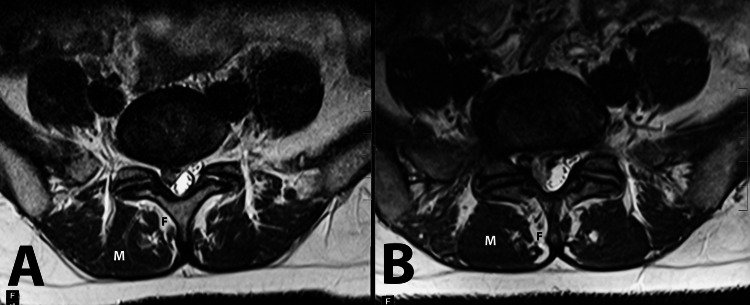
Axial T2-weighted MRI images of Patient 2 at initial presentation (A) and three years later. Note the progression of fatty infiltration (black letter F) and multifidus muscle atrophy (white letter M).
